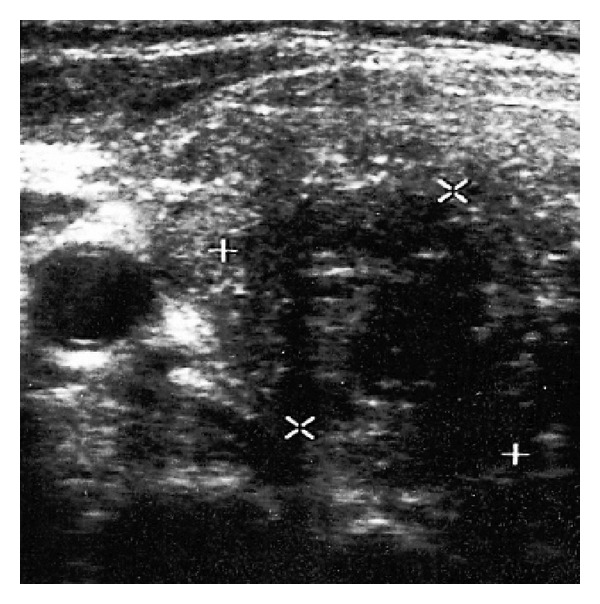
Ultrasound finding of parathyroid carcinoma (transverse)—an intrathyroid, nonhomogeneous, fibrotic mass in the right lobe.
